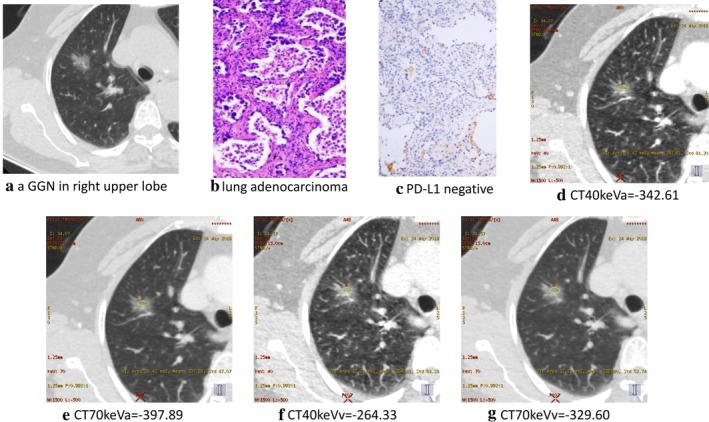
A 69‐year‐old female with lung adenocarcinoma had negative PD‐L1 expression on (a) conventional CT, (b) pathological section, (c) PD‐L1 expression, (d) CT40keVa, (e) CT70keVa, (f) CT40keVv and (g) CT70keVv.
